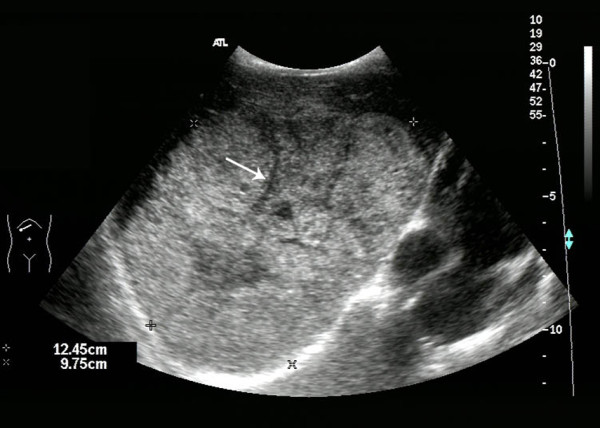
HBL in the right lobe of the liver in a 2-year-old girl. A grey scale ultrasound image revealed the septa within the lesions (arrow).
